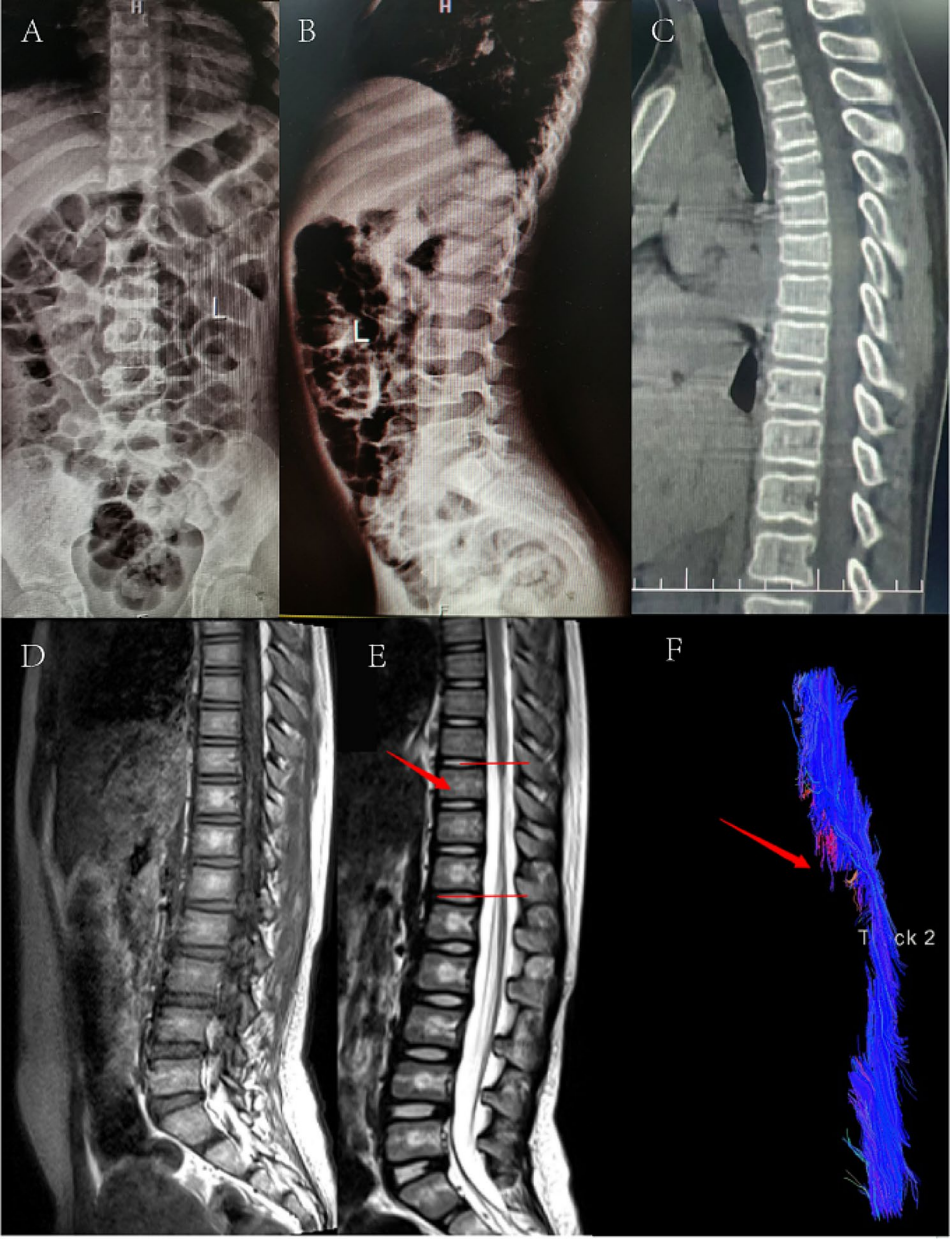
A 10-year-old female patient presented with paralysis after dance backbend practice. X-ray (A, B) and CT (C) scans did not detect any fractures or dislocations. No abnormmality was found on T1-weighted MRI (D), T2-weighted MRI (E) showed localized spinal cord thinning at the T10-12 vertebral level. DTI fiber reconstruction images (F) revealed local fiber areas and thinning of fiber bundles
